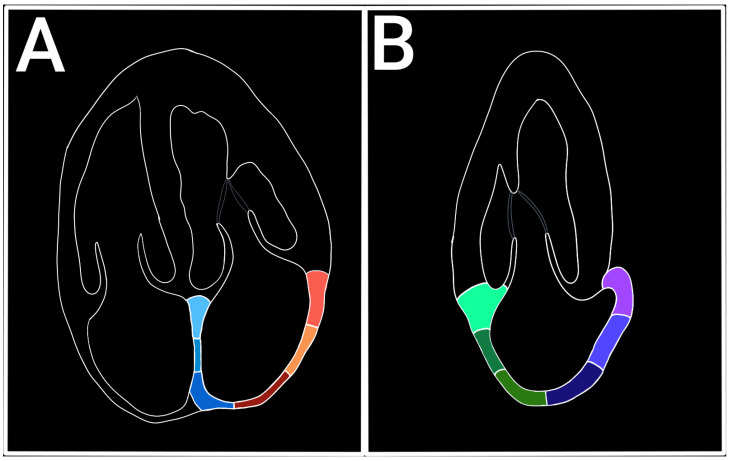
Segmentation of the left atrium. (A) Apical four chambers. (B) Apical two chambers.
